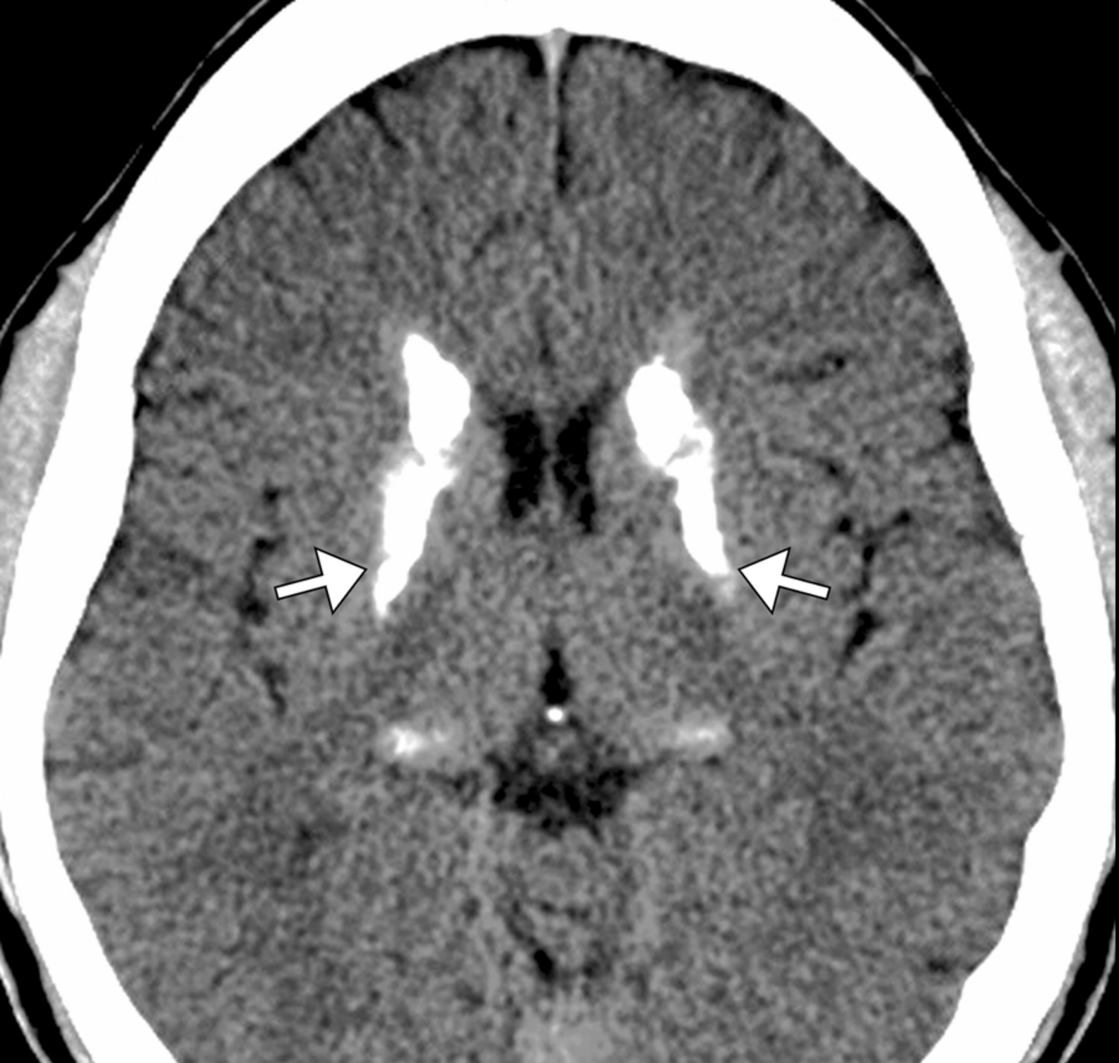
Axial CT of the brain showing bilateral dense calcification (arrows) of the basal ganglia.
